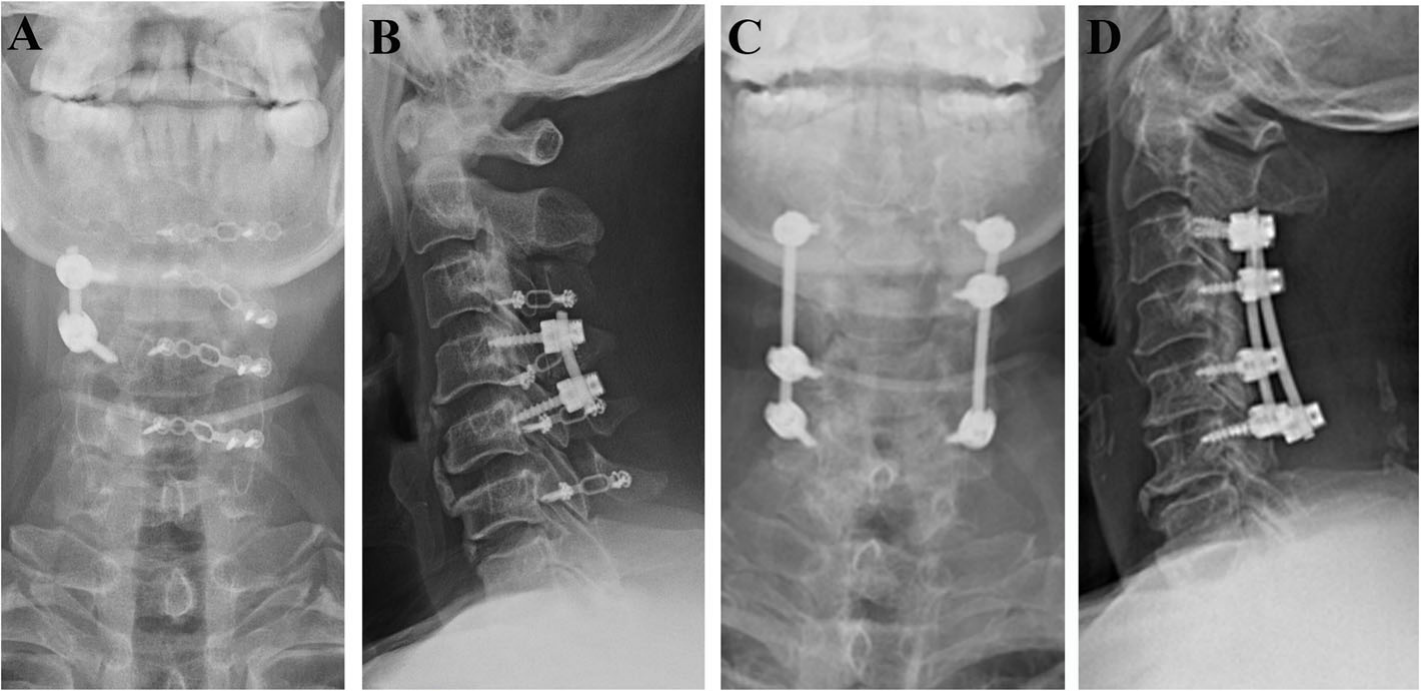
Postoperative cervical radiography of patients treated with laminoplasty and fixation at the unstable segment (a and b) and laminectomy and fusion (c and d)
